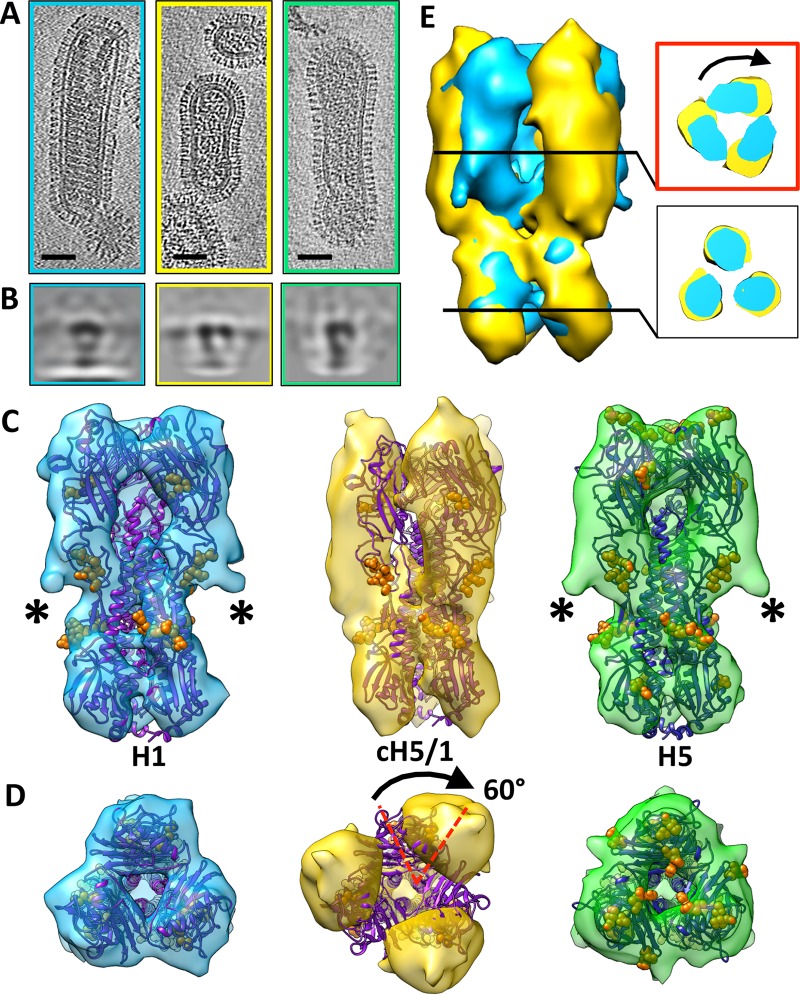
Cryo-EM density maps reveal differences in morphology of the chimeric HA compared to H1 and H5 HA. For all panels, H1 HA, cH5/1 HA, and H5 HA are indicated by cyan, gold, and green, respectively. (A) Tomographic slices of single pH1N1, cH5/1N1, or H5N1 viruses are shown from left to right, respectively. (B) Projection views of slices through averaged subtomogram volumes for (from left to right, respectively) H1, cH5/1, or H5 HA show a side view of each HA. (C and D) Isosurface representations of density maps are shown in side (C) and top (D) views, respectively. Density maps are fitted with X-ray coordinates for H1 HA (purple) or H5 HA (dark blue). Glycosylated residues are represented as solid orange spheres. Corresponding density within the density maps is marked by asterisks. (D) The mismatch between the X-ray coordinates and the cH5/1 HA density map is indicated with a black arrow. (E) An overlay of the cH5/1 and H1 HA maps aligned by the stalk region is shown. Cross-sectional views of the head and stalk regions (boxed insets) show the head region misalignment of the two maps (indicated by a black arrow).
